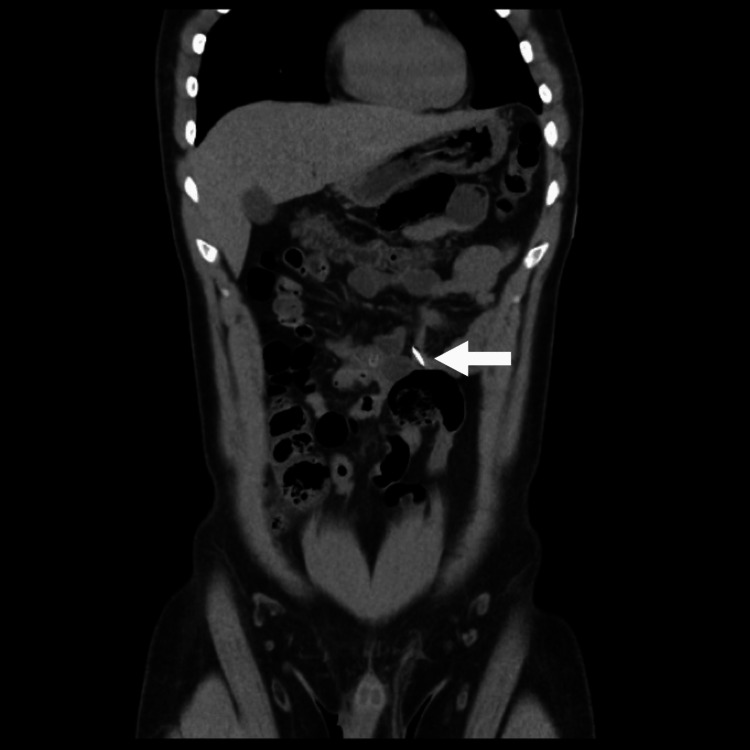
Computed tomography scan showing needle-shaped body in mid-jejunal loops.
